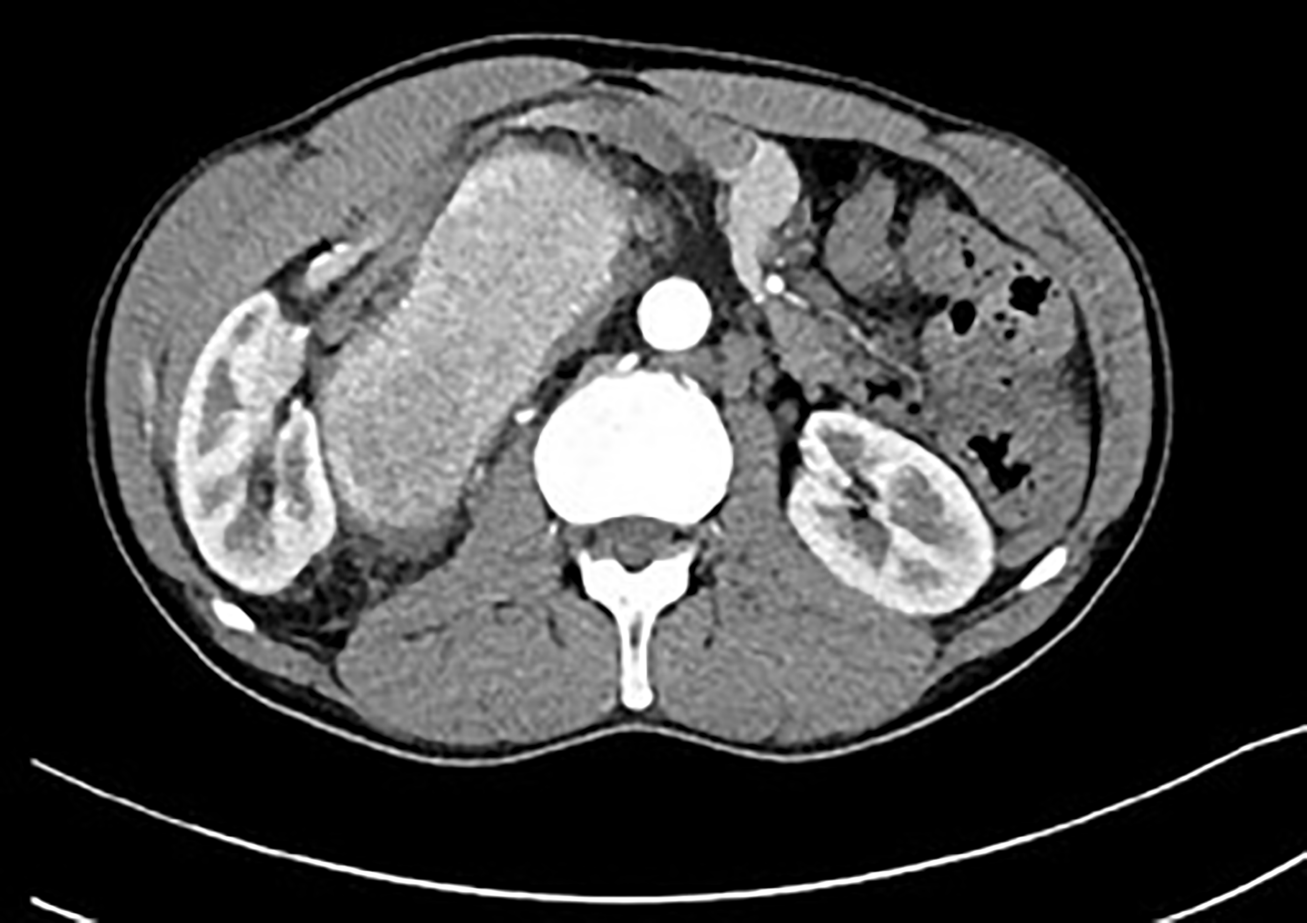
Postcontrast CT of unicentric form of Castleman disease. Markedly enhanced mass in right retroperitoneal space displacing right kidney.
